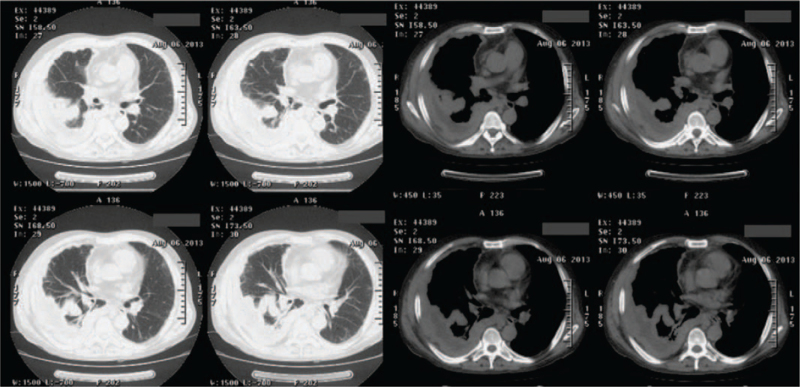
Irregular pleural thickening, little hydrothorax, and a large mass occupying the middle and inferior lobe of right lung are visible in the nonenhanced CT imaging of chest. CT = computed tomography.
